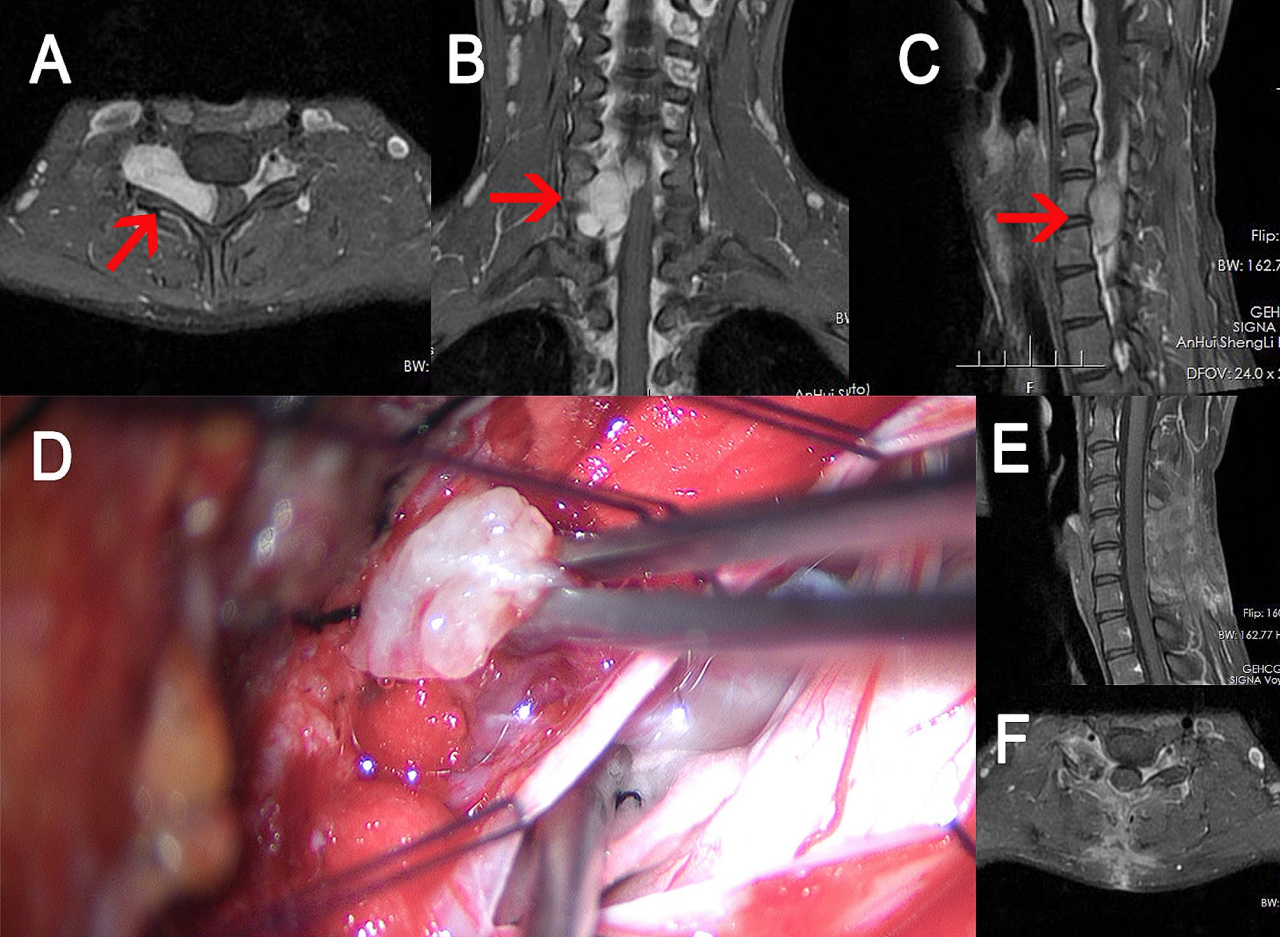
The data of case 2. A, B, and C: preoperative MR images. The tumor (red arrow) was located at the C6-7 level. D: images during the operation. Pulling the tumor through one incision combined with pushing through another incision. E and F: MRI performed two months after the operation
